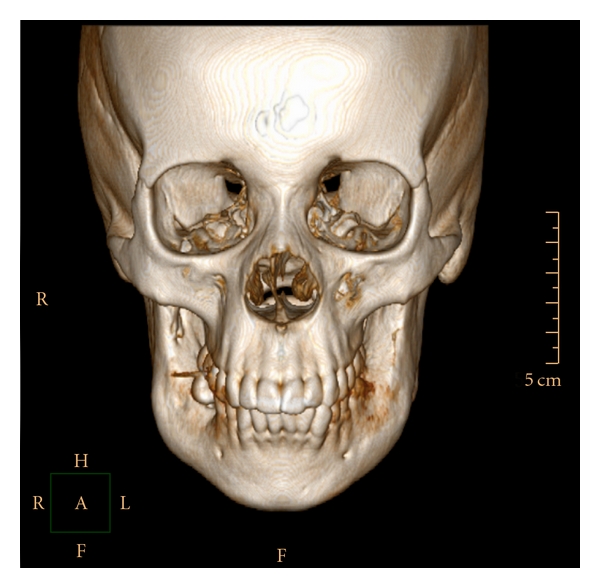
3D reconstruction of the CT scan images showing absence of depression and erosive areas at the mandibular body and retromolar region.
